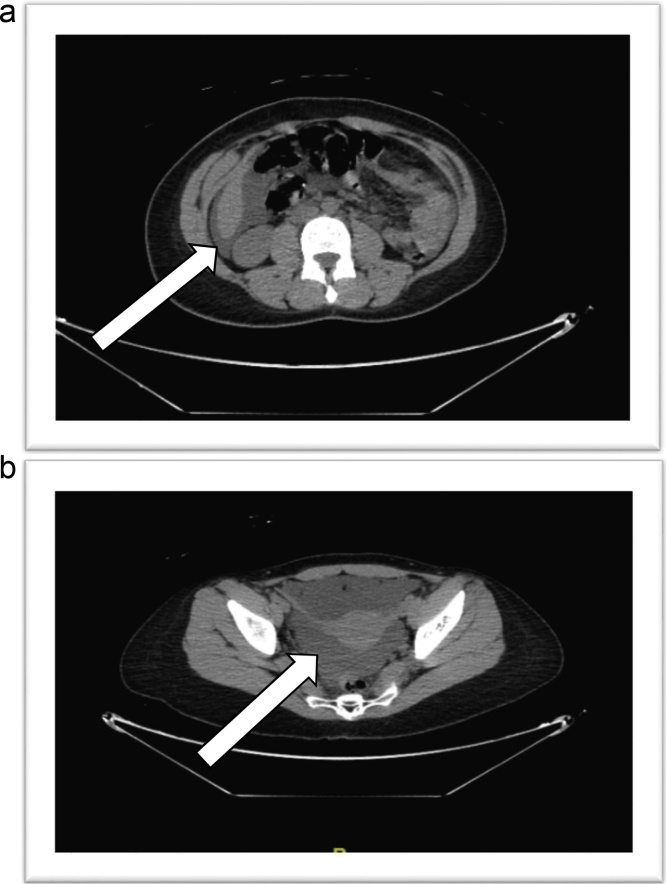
A: CT abdomen showing simple fluid density (urine) in perihepatic space and hepatorenal fossa. B: CT pelvis showing simple fluid density (urine) surrounding the uterus.
